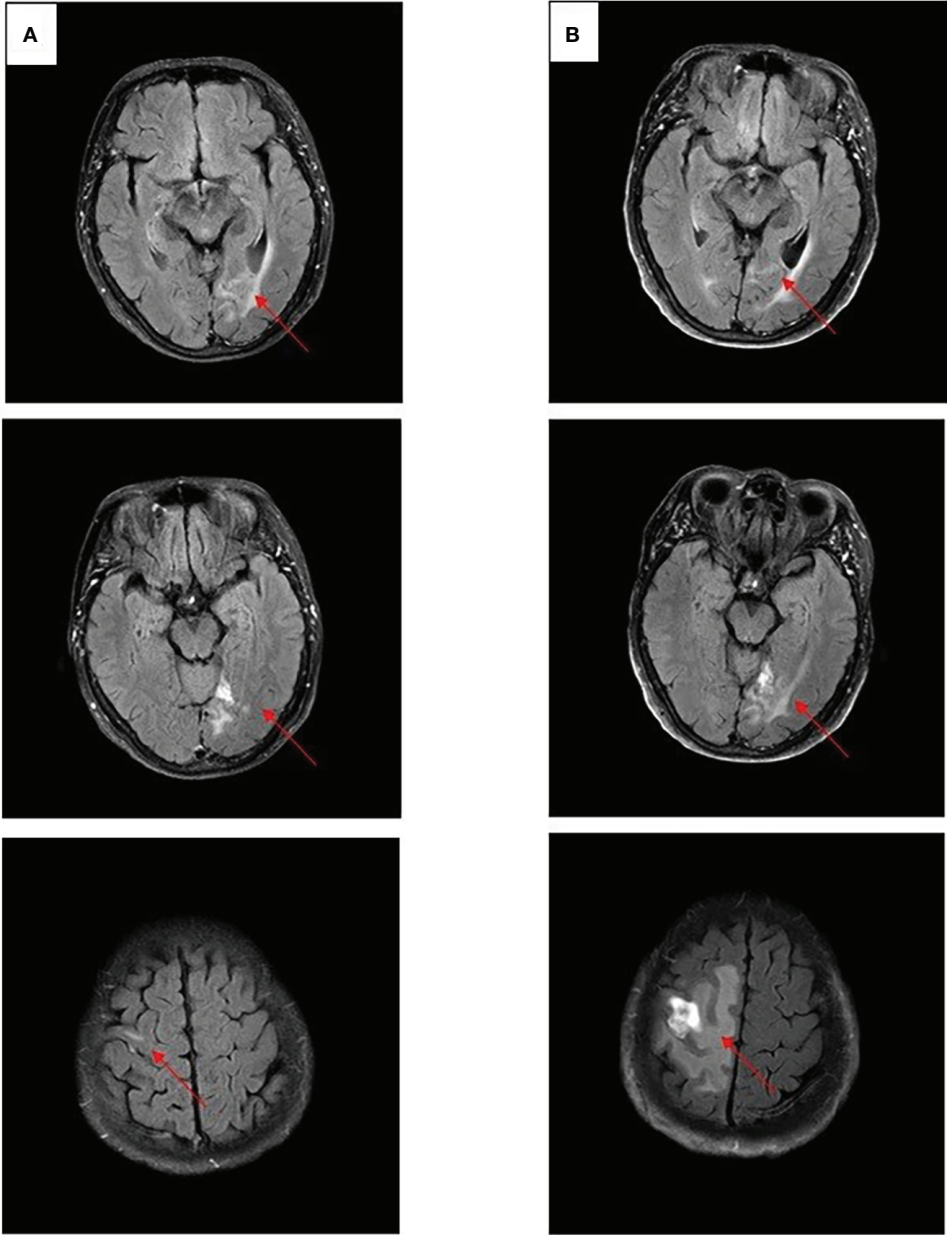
Craniocerebral MRI about clinical response to dacomitinib therapy at different times. (A) Brain metastases still PR after 12 months. (B) Brain metastases PD after 17 months.
